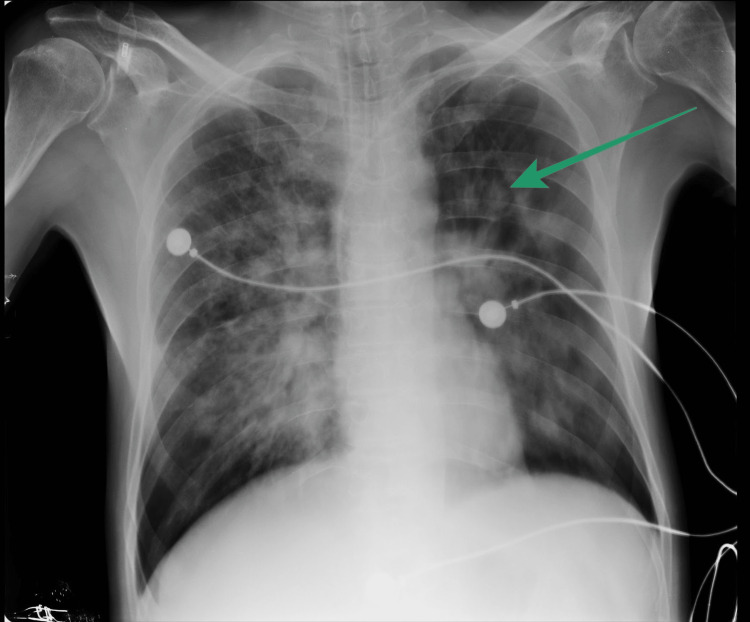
A plain radiograph of the chest suggestive of tuberculous cavitation (anteroposterior view).
